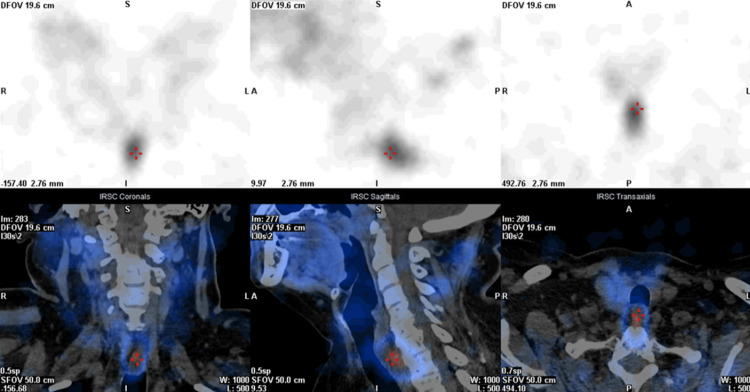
99mTc-sestamibi SPECT/CT indicating a MIBI-avid focus in the midline posterior cervical region (red crosshairs) suggestive of an ectopic parathyroid adenoma. MIBi: Tc-99m methoxyisobutylisonitrile; Tc: technetium; SPECT: single-photon emission computed tomography
